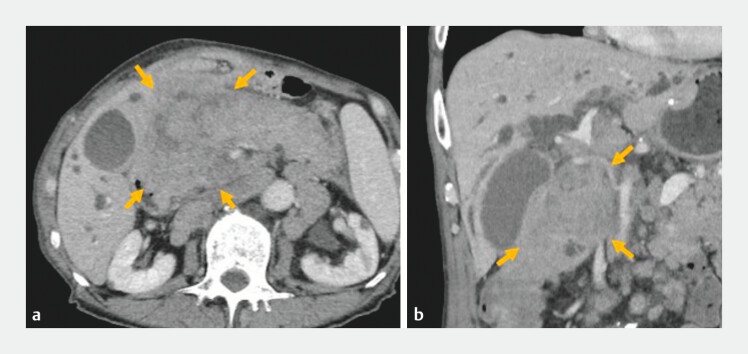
Computed tomography showing intrahepatic bile duct dilation and duodenal obstruction because of the recurrent tumor (arrows).
